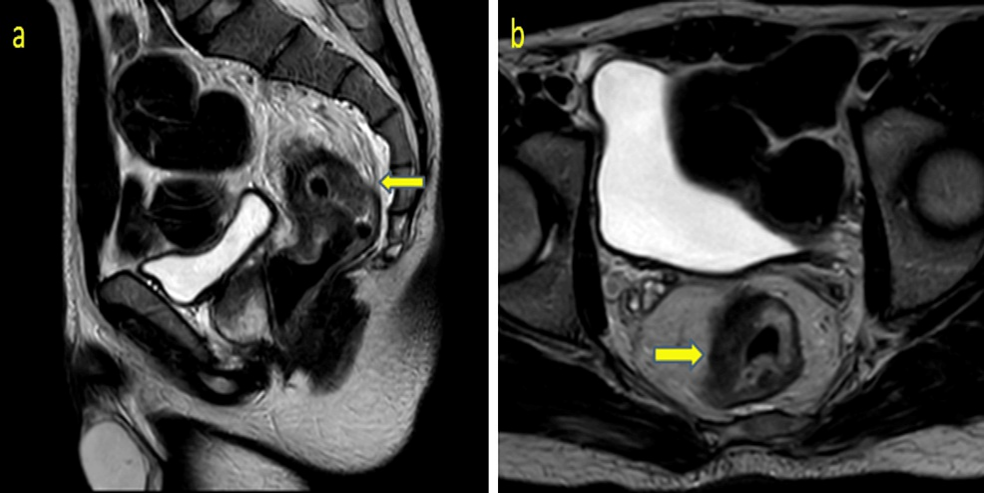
Sagittal (a) and axial (b) pelvis MRI scan T2 weighted images showed diffuse wall thickening of the rectum with mesorectal fat involvement (yellow arrows).
